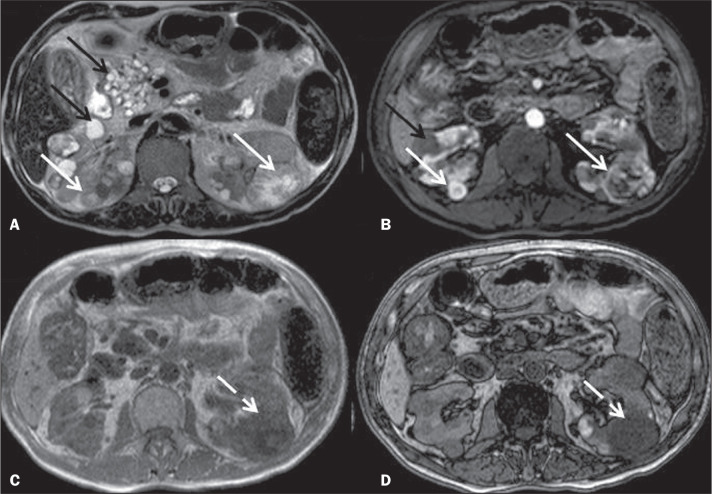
Axial MRI. T2-weighted sequence (A) and T1-weighted sequence
